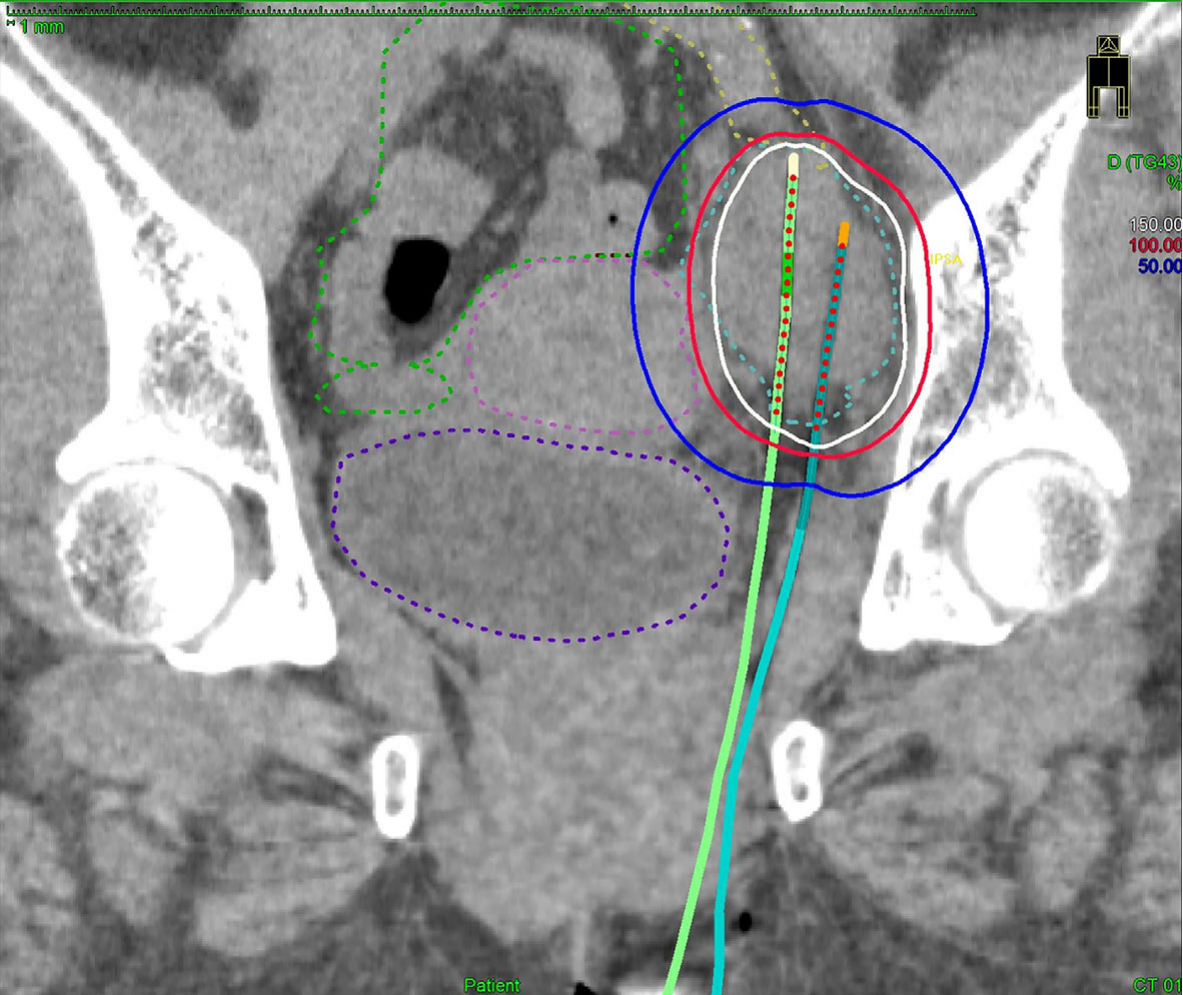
A coronal CT image of the bulkiest obturator node (blue dashed line), uterus (pink dashed line), bowel (green dashed line), external iliac vessel (yellow dashed line), bladder (purple dashed line) receiving 50% (blue lines), 100% (red lines), and 150% (white lines) of the prescription dose (6 Gy) from two implanted needles.
